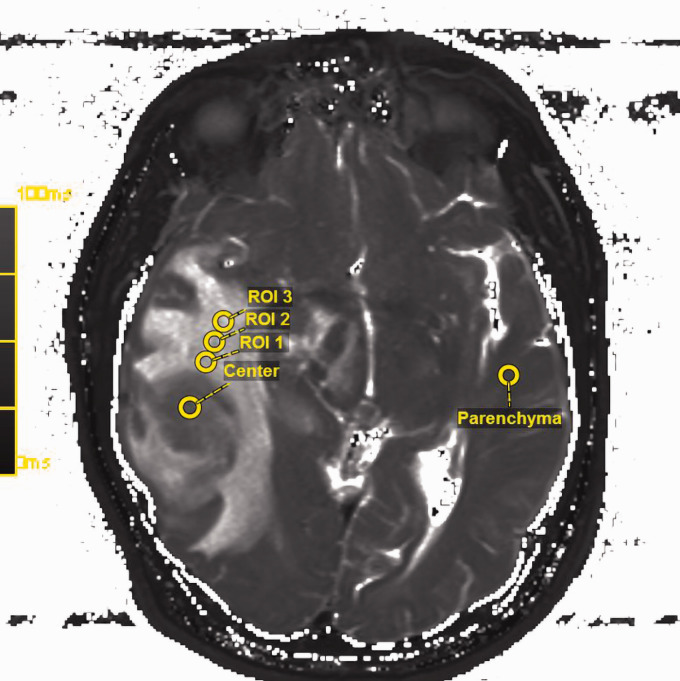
Region of interest (ROI) placement in the raw T2 map in accordance with the fluid-attended inversion recovery (FLAIR) sequences. The slice with the largest diameter of peritumoral T2-weighted/FLAIR hyperintensity was chosen, the anatomical centre of the tumour was delineated. Another ROI was placed in the healthy- appearing white matter of the contralateral lobe using an image processing program to ensure reliability of the measurements. The spatial T2 value distribution across the tumour radius was measured at four different locations from the tumour centre to the outer infiltration zone by the placement of three further ROIs.
